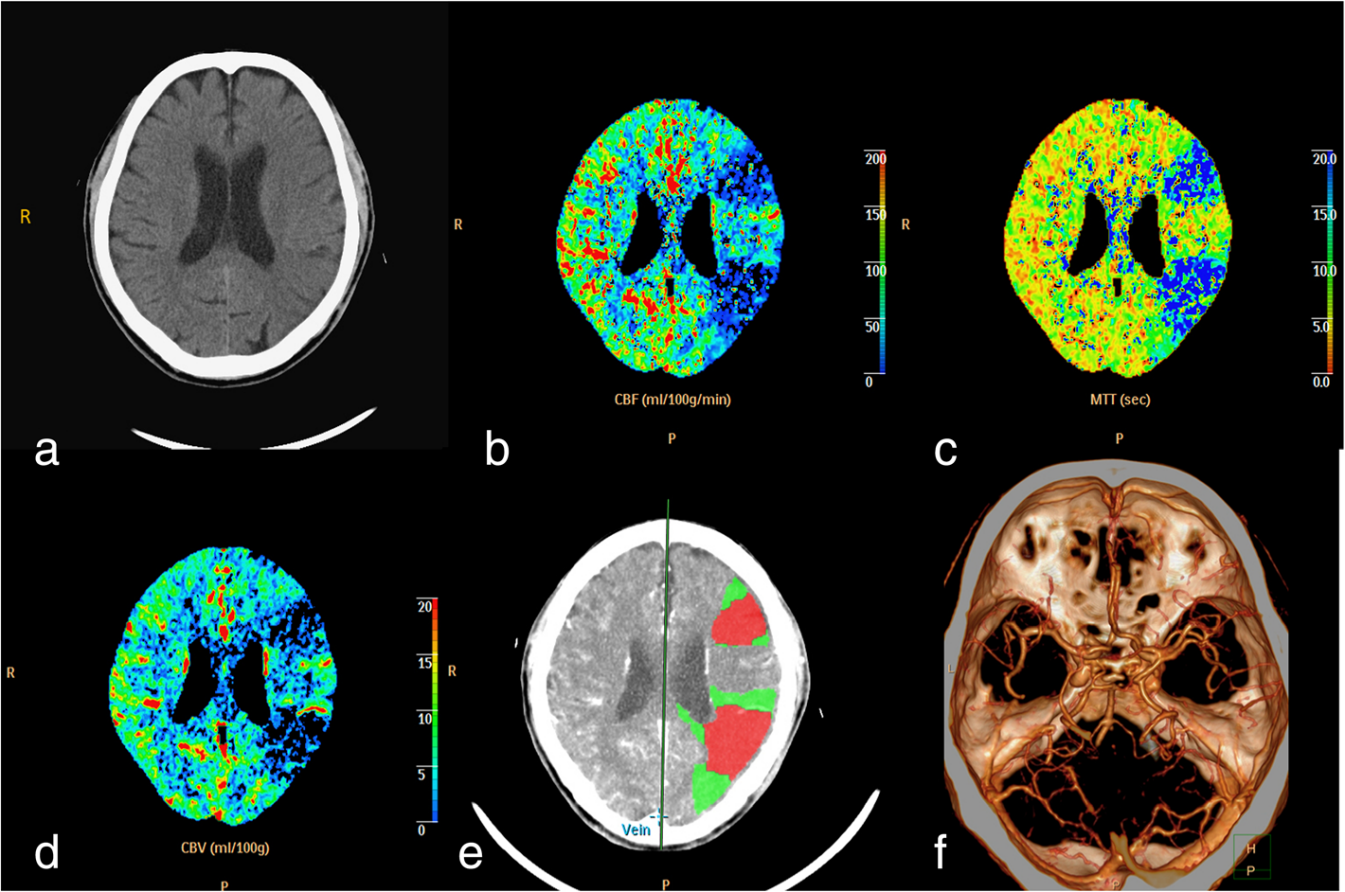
Brain CT and perfusion imaging-derived maps obtained in the emergency department. a: Pre contrast image showed no haemorrhage or obvious hypodense region. b: Cerebral blood flow (CBF). c: Mean transit time (MTT). d: Cerebral blood volume (CBV). e: CT perfusion (CTP). Core-penumbra colour map with estimated core and penumbra areas, highlighted in red and green, respectively. CTP shows a large deep core and penumbra regions over the left middle cerebral artery territory. f: CT angiography (CTA) showed no obvious vascular lesion on the main trunk of the intracranial arteries
